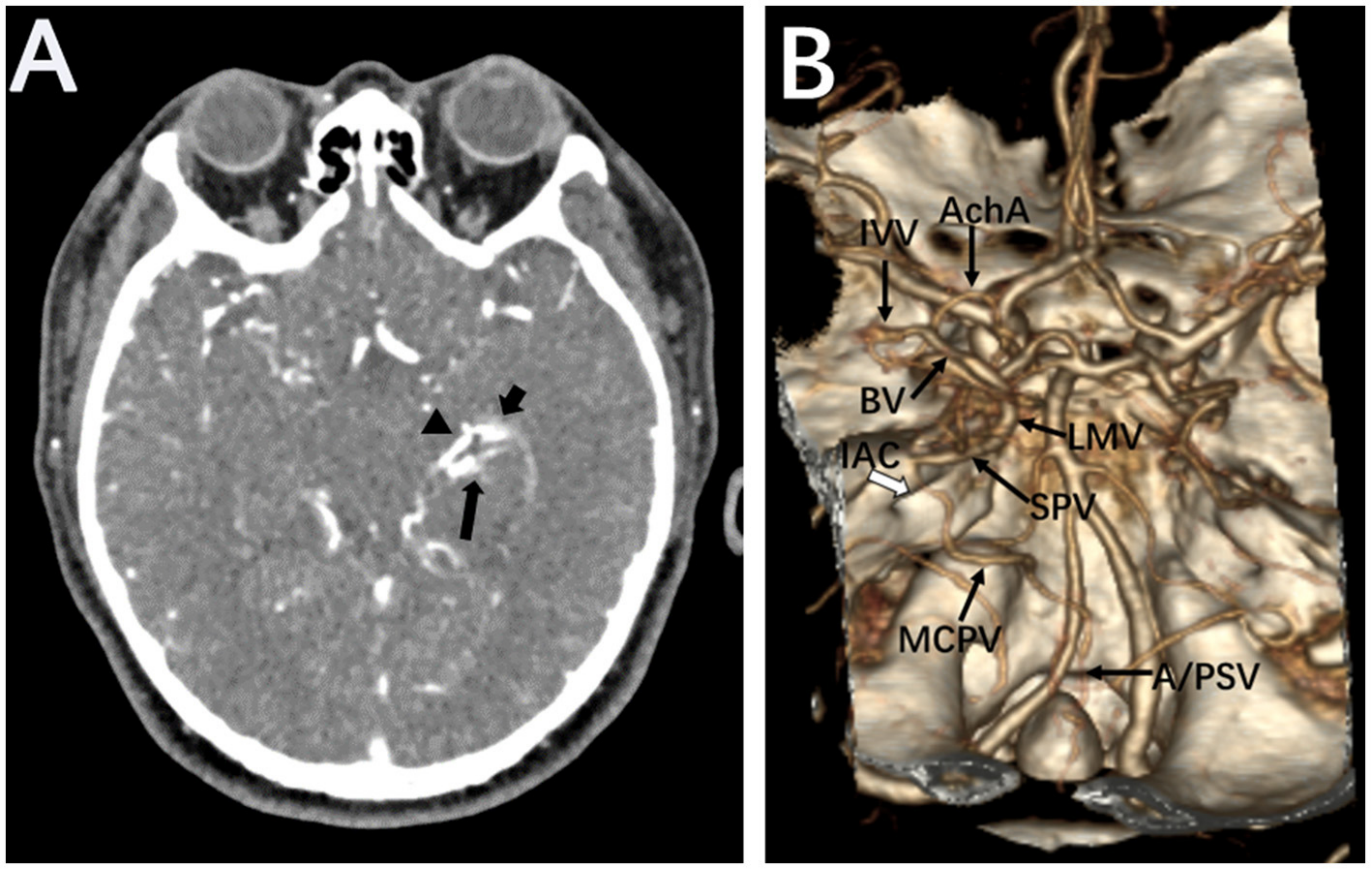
Brain CTA. Source images of brain CTA showed a large abnormal vessel (arrowhead) connecting to a vascular cluster close to the inferior horn of the left lateral ventricle (short arrow). The left posterior cerebral artery was also disclosed (long arrow) (A). Volume reconstruction of CTA revealed the supplied artery and drainage veins of the micro-AVM on the choroidal fissure (B). anterior choroidal artery, AchA; inferior ventricular vein, IVV; basal vein of Rosenthal, BA; lateral mesencephalic vein, LMV; superior petrosal vein, SPV; middle cerebellar peduncle vein, MCPV; anterior and posterior spinal veins, A/PSV; internal auditory canal, IAC.
